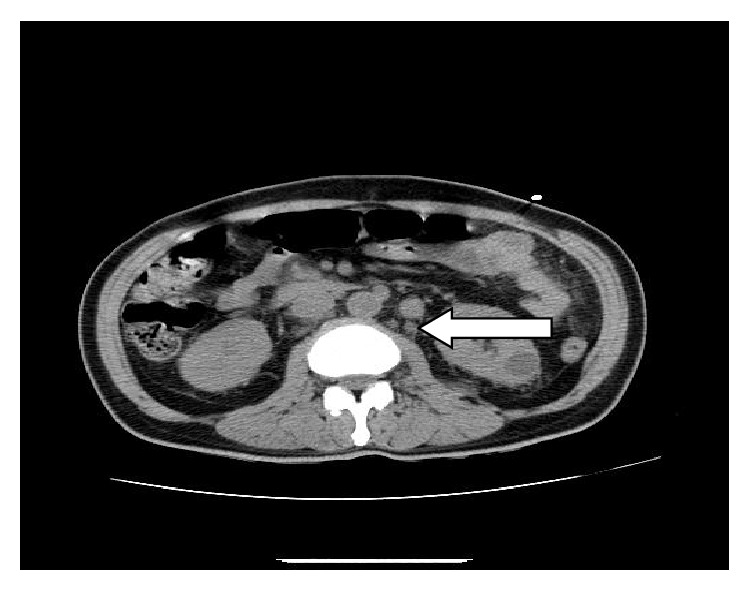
CT of abdomen without contrast showing lymphadenopathy.
